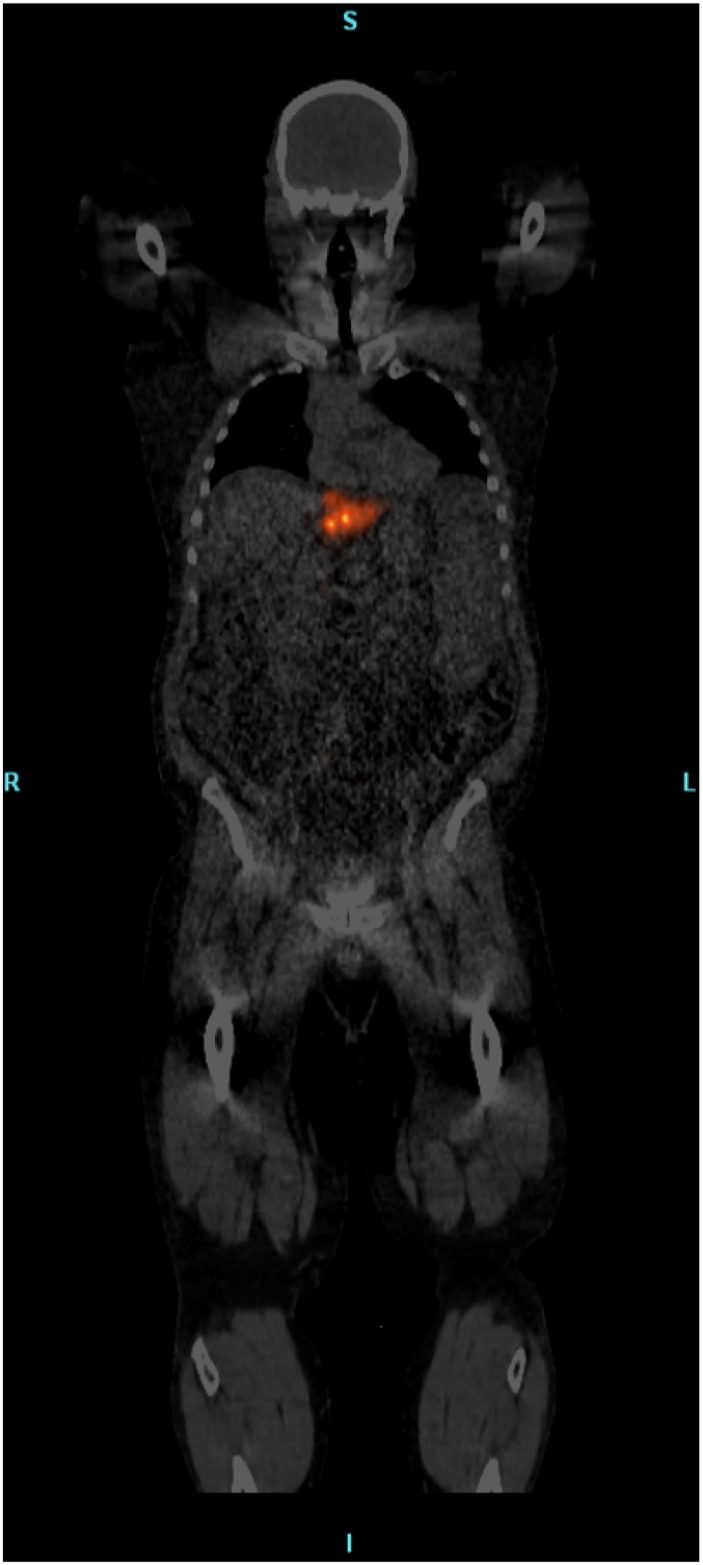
Whole-body fused PET/CT image showing only focal activity at the site of treatment and no widespread aggregation of free Y90 detected.
